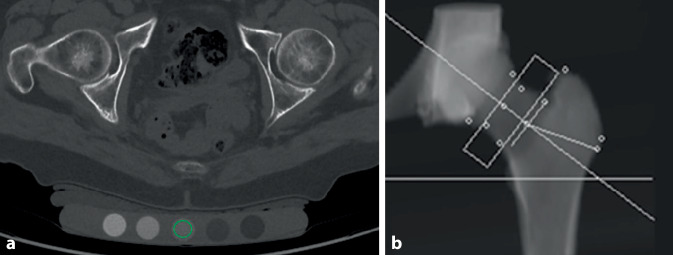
CTXA („computed tomography X‑ray absorptiometry“): Planung der trabekulären Bestimmung des Knochenmineralgehalt im CT-Schnittbild (a, b). Im Bild *links*
